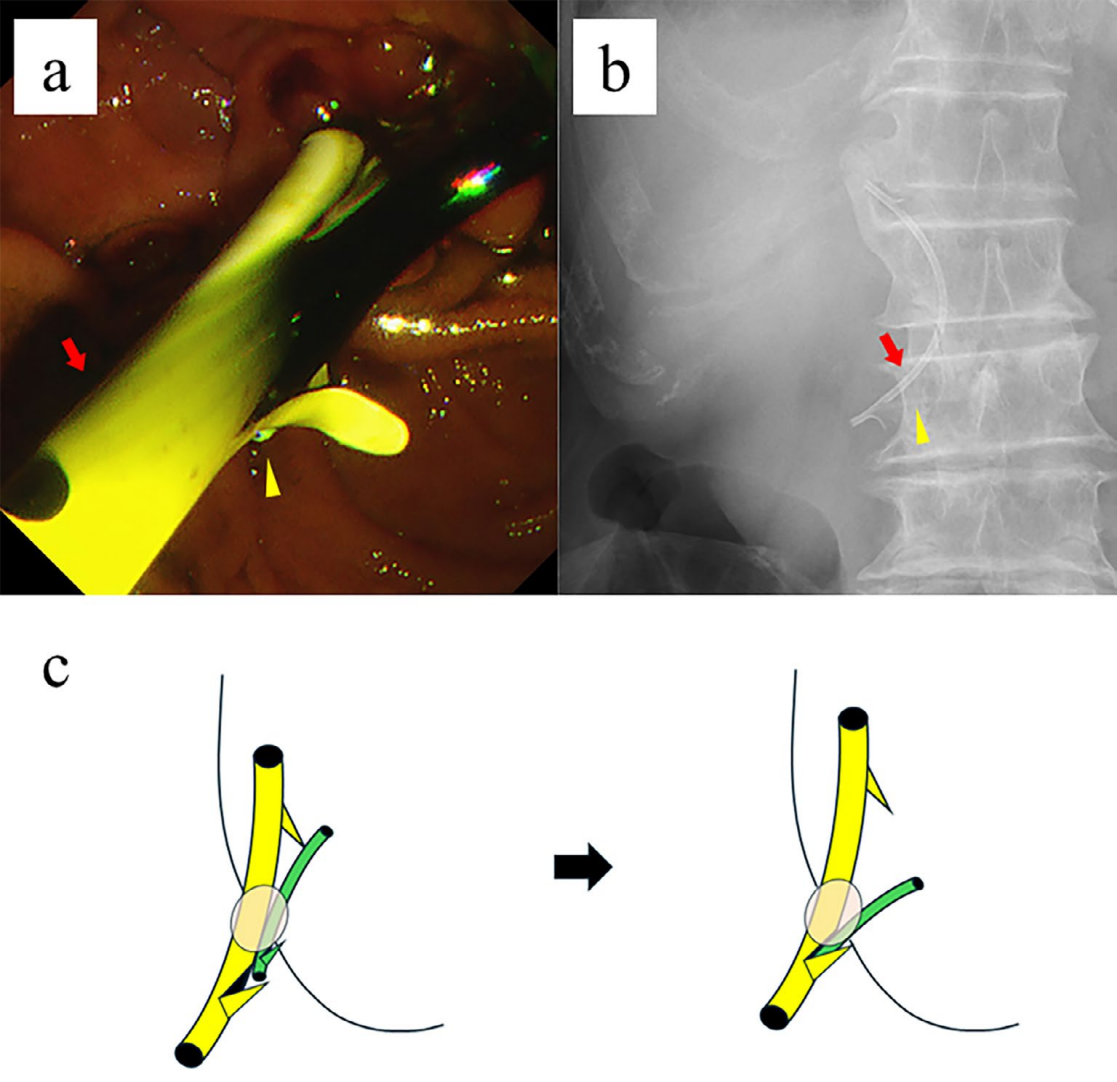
(a) Endoscopic image taken during endoscopic retrograde cholangiopancreatography (ERCP) showing the original position of the stents. The yellow arrowhead shows the distal end of the pancreatic stent and the red arrow shows the biliary stent. (b) Abdominal radiography performed the day after stent placement showing migration of the pancreatic stent into the biliary stent. The yellow arrowhead shows the pancreatic stent and the red arrow shows the biliary stent. (c) Pictorial representation of the migration of the pancreatic stent into the biliary stent through a flap in the biliary stent.
